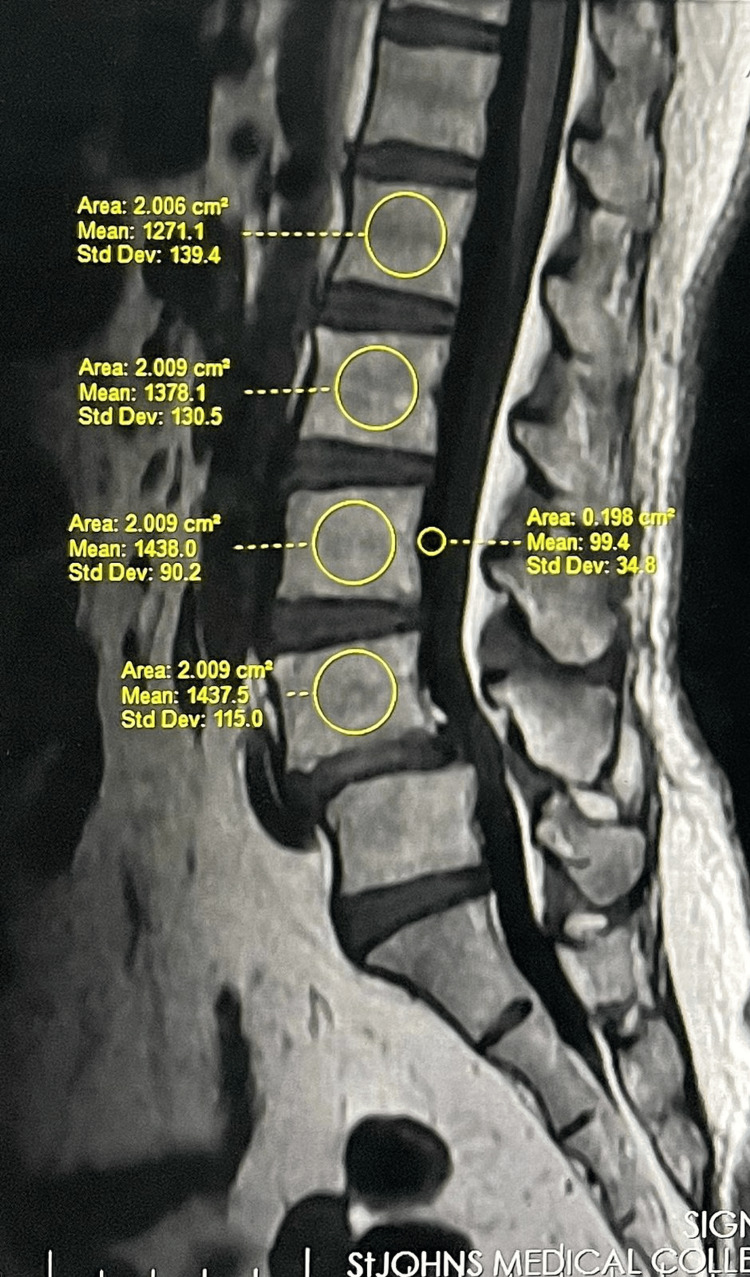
MRI-based vertebral bone quality (VBQ) score derivation from routine T1-weighted sagittal non-contrast sequences.
